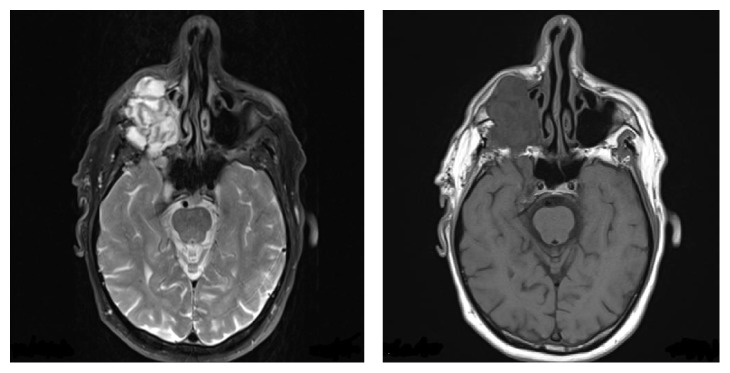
Axial MRI showing lobulated mass in the right maxillary antrum extending anteriorly into the subcutaneous tissue of the cheek and posteriorly into the infratemporal fossa.
